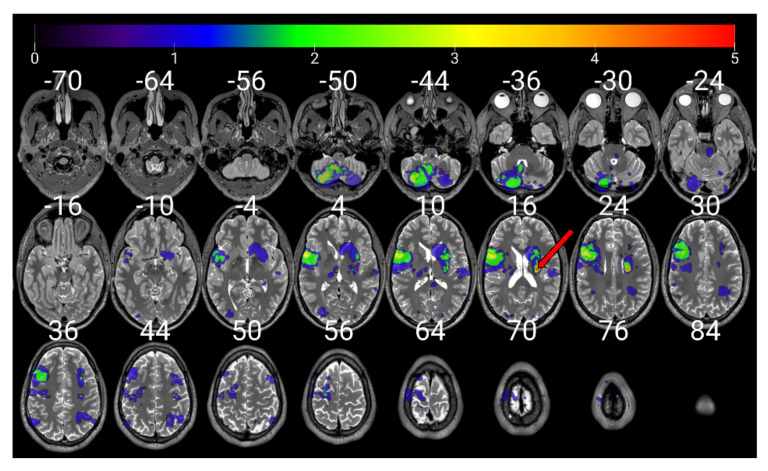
Superposition of the individual MRI lesion masks. Mosaic display of axial slices presented in neurological convention (left hemisphere on the left). The red arrow is pointing at the only cluster where at least 5 patient’s lesions overlapped. The color bar symbolizes the number of patients’ lesions overlapping from 0 to 5.
